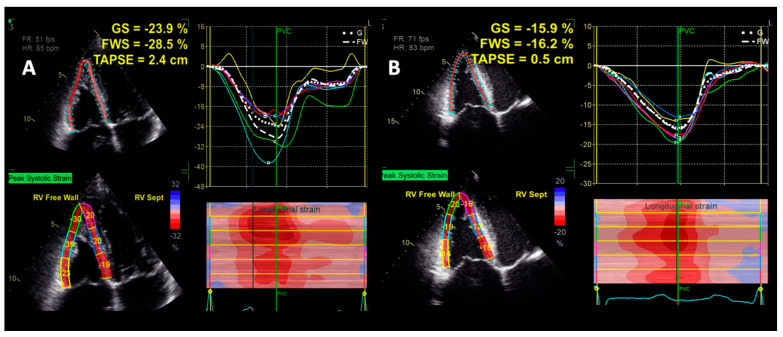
Right ventricular functional assessment by speckle–tracking echocardiography in a patient with (A) normal right ventricular longitudinal function, and (B) right ventricular longitudinal dysfunction. Abbreviations: FWS, free wall strain; GS, global strain; other abbreviations as in Figure 1.
